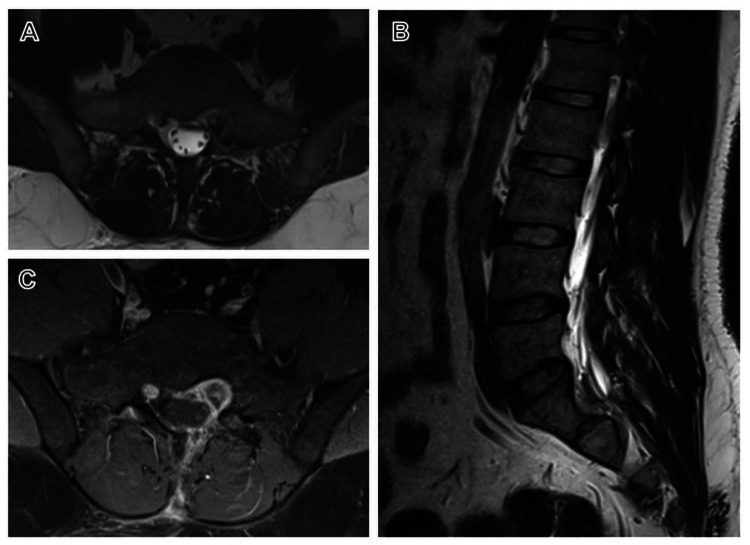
MRI of the lumbosacral spine T2 axial (A) and T2 sagittal (B) images show a small residual lesion in the left S1 foramen. Post-contrast T1 image (C) demonstrates peripheral enhancement of the lesion.
